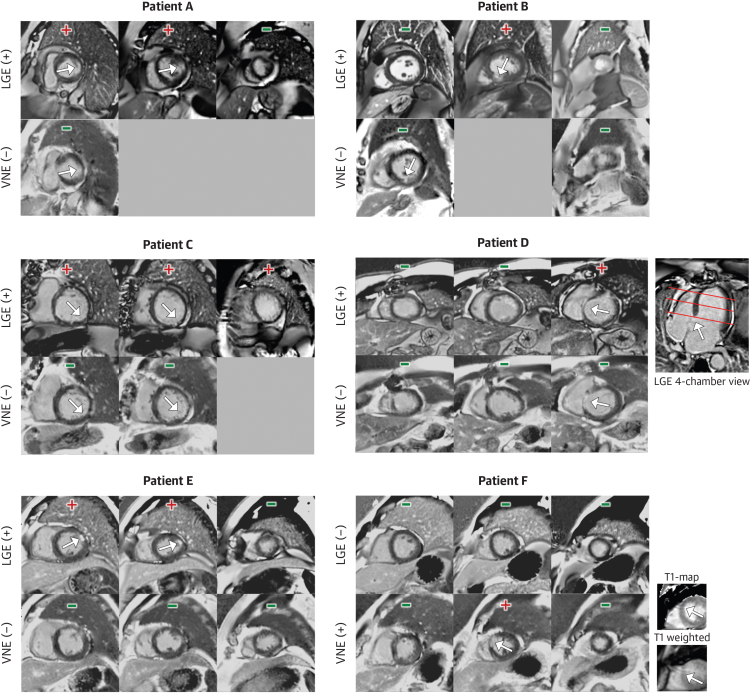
All 6 Cases With Discrepant LGE and VNE Scores for the Presence or Absence of MI Returned by Blinded, Independent Multiobserver Assessment Plus signs denote scored as infarct or possible infarct; minus signs denote scored as no infarct. Gray panels indicate missing image slices. (A) Discrepancy attributed to missing VNE slices. The single available VNE slice showed a small area of enhancement in the basal lateral wall, similar to LGE, but the VNE observer did not have the subsequent midventricular slice also showing a lateral wall scar to help diagnose a case of lateral MI. (B) Discrepancy attributed to missing VNE slice. The corresponding LGE slice showed an inferoseptal infarction. (C) Discrepancies attributed to interobserver variability in scoring MI. Both LGE and VNE showed thin subendocardial enhancement in the inferolateral wall but were interpreted differently. The LGE scorer would have had the entire short-axis stack and also long-axis LGE views to help recognize the presence of MI. (D) Left ventricular outflow tract on LGE basal slice was misinterpreted as scar, whereas the VNE scorer had attributed the enhancement to outflow tract partial volume effect. This was later confirmed on the 4-chamber long-axis LGE image. VNE and LGE images had good visual-spatial agreement. (E) A false negative VNE case: a small MI scar was missed on VNE. (F) A false positive VNE case: the VNE signal was later attributed to fat off-resonance artifact on the corresponding T1-map and T1-weighted images. Abbreviations as in Figure 1.
